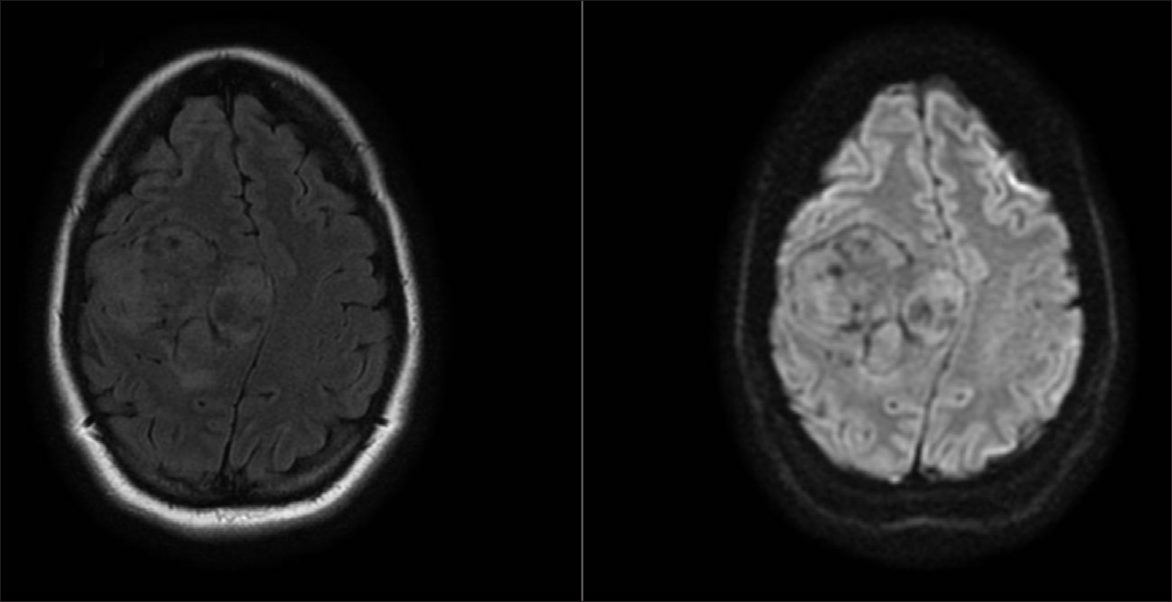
T2FLAIR and DWI show a midline shift but no diffusion restriction.
